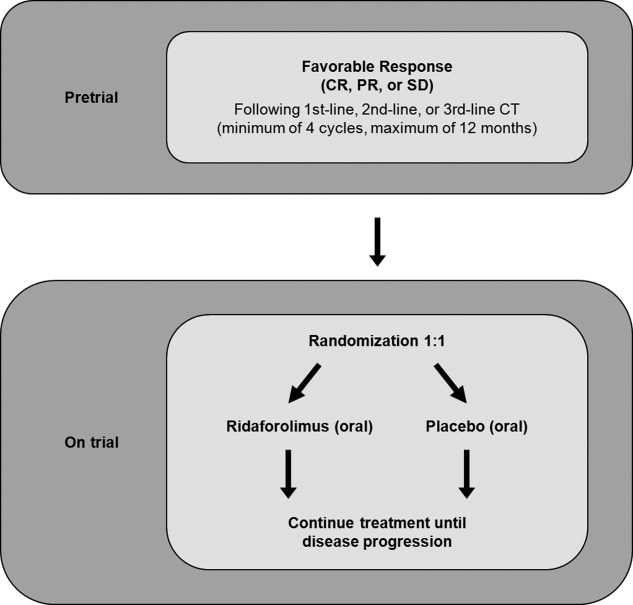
The *S*arcoma M*u*lti-*c*enter *C*linical *E*valuation of the *E*fficacy of Ri*d*aforolimus (SUCCEED) study scheme is shown. CR indicates complete response; PR, partial response; SD, stable disease; CT, chemotherapy.
